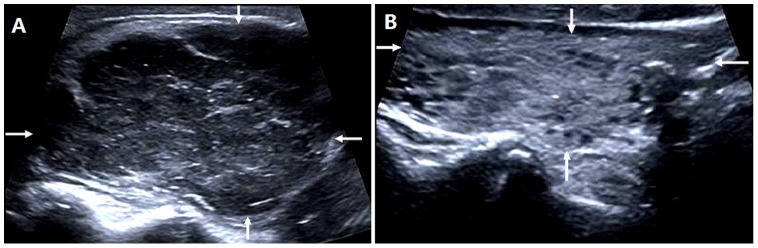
A 67-year-old male patient with non-diffuse PTL. (A)The longitudinal sonogram shows that PTL was limited to the right thyroid lobe (arrows). (B) The longitudinal sonogram shows a heterogeneous echotexture of the left thyroid lobe (arrows).
